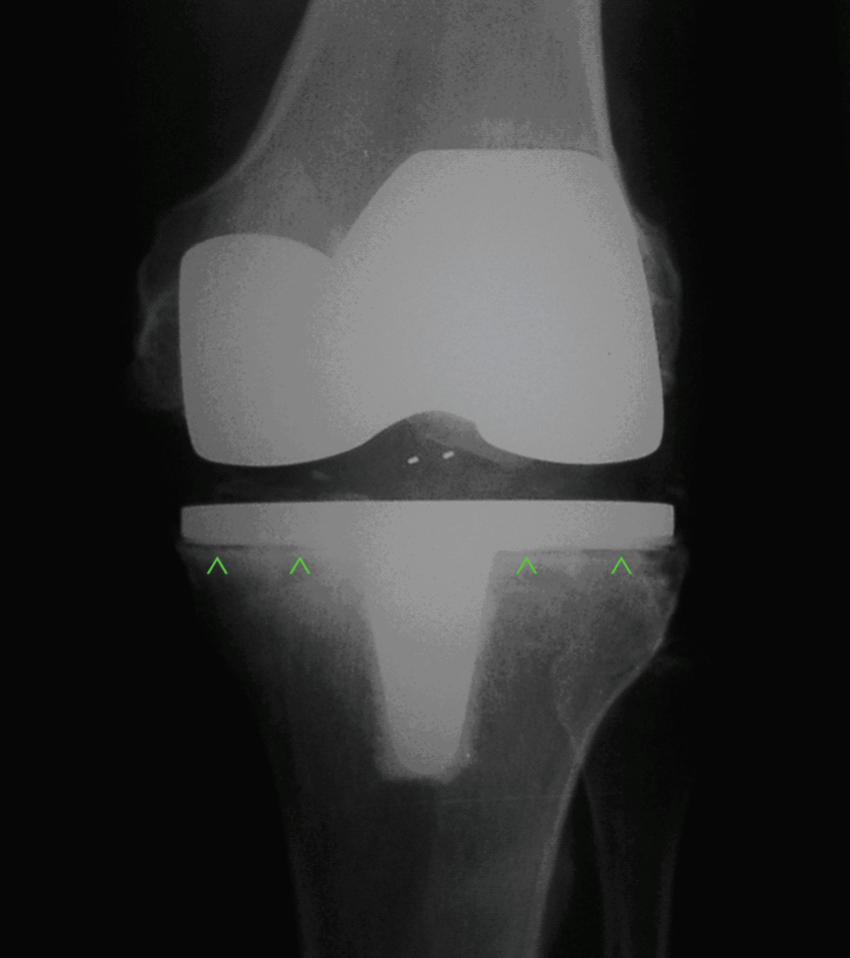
Anterior-posterior X-ray of a left Low-contact-stress total knee prosthesis (with cemented tibia) in a patient with continuous moderate knee pain with tibial stress shielding in zones "1", "2", "3", and "4", according to the classification of Ewald et al. [20].
